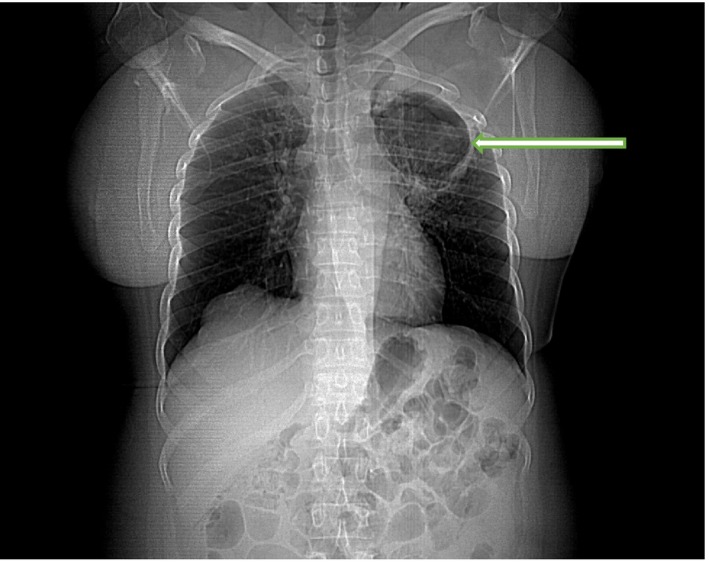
Chest CT scan showing a thick‐walled cavity in the left upper lobe.
